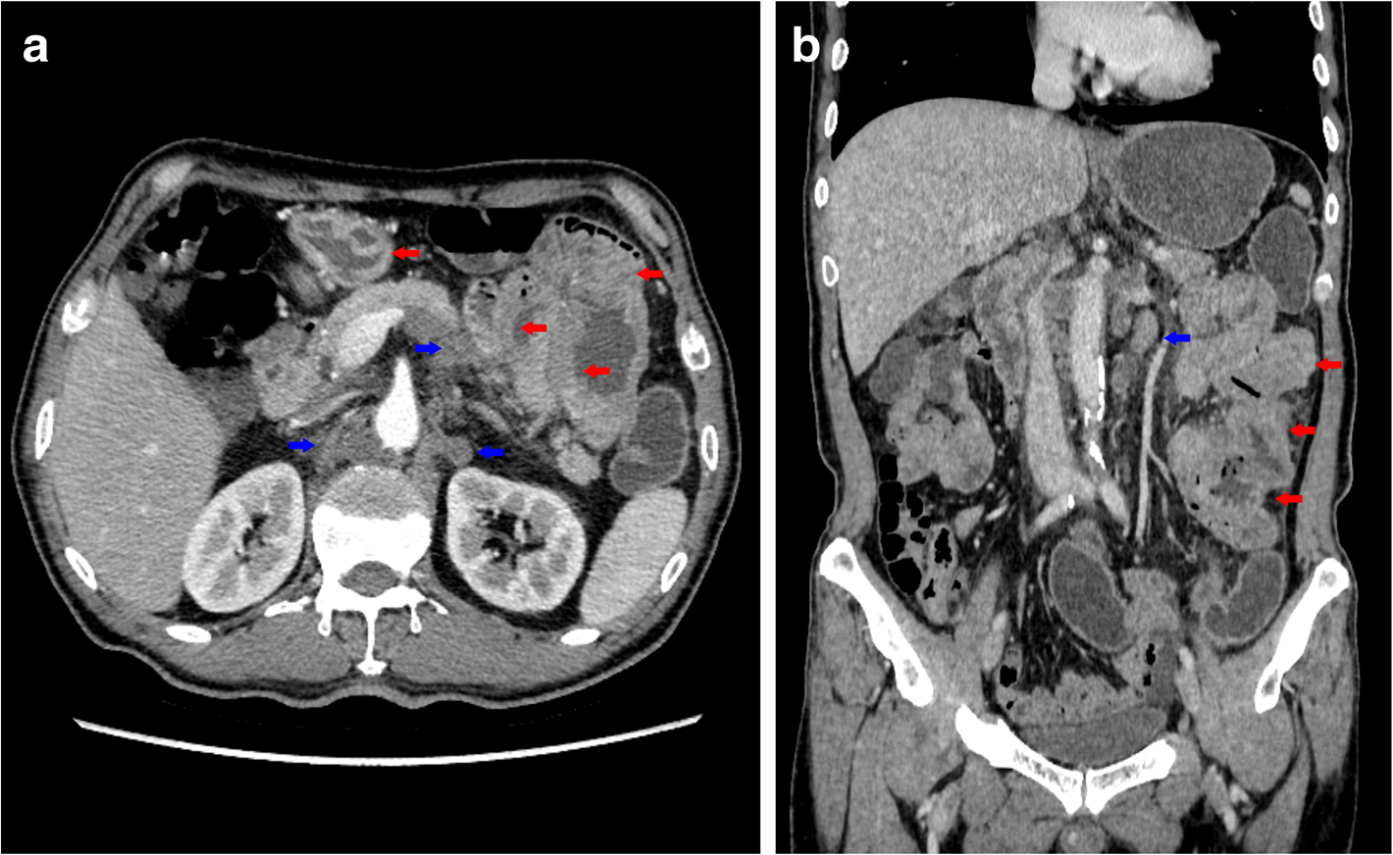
Abdominal CT. a-b Multiple thickened small intestinal walls (red arrow) in the left abdomen and multiple enlarged lymph nodes (blue arrow) in the surrounding and posterior peritoneum
